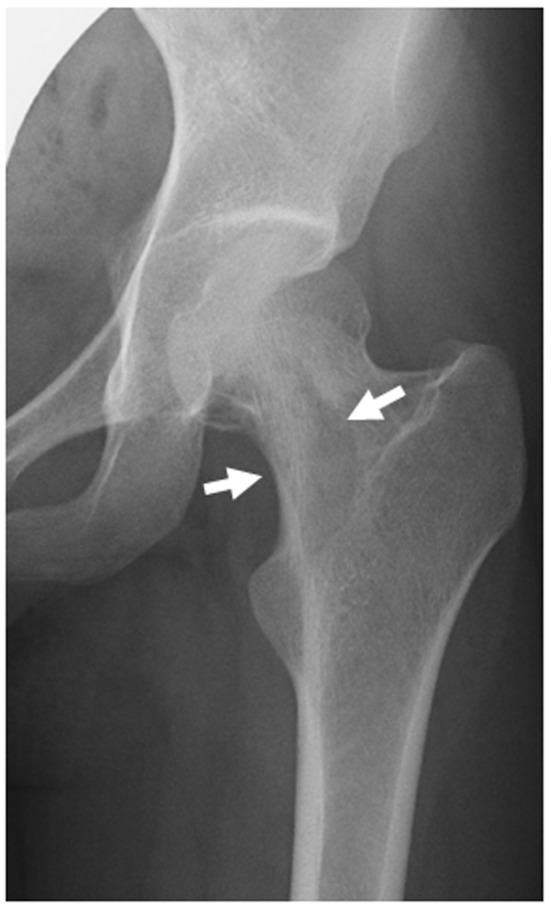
Radiographic observations at the time of the initial consultation showing an osteolytic lesion with an osteosclerotic change to the femoral neck (arrows).
